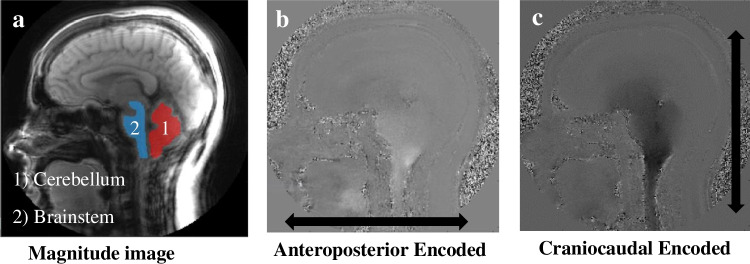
Example midsagittal DENSE MR image set: a magnitude image, b phase image with displacement encoded in the anterior–posterior direction, and c phase image with displacement encoded in the cranial-caudal direction. Black arrows show the displacement encoding direction
